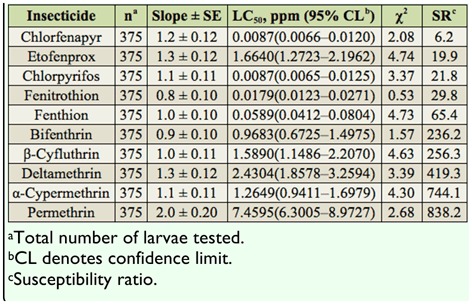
Summary of toxicities of 10 insecticides to third instars of the DG-CT strain of *Culex tritaeniorhynchus* based on the 24 hr exposure contact mortality bioassays.
